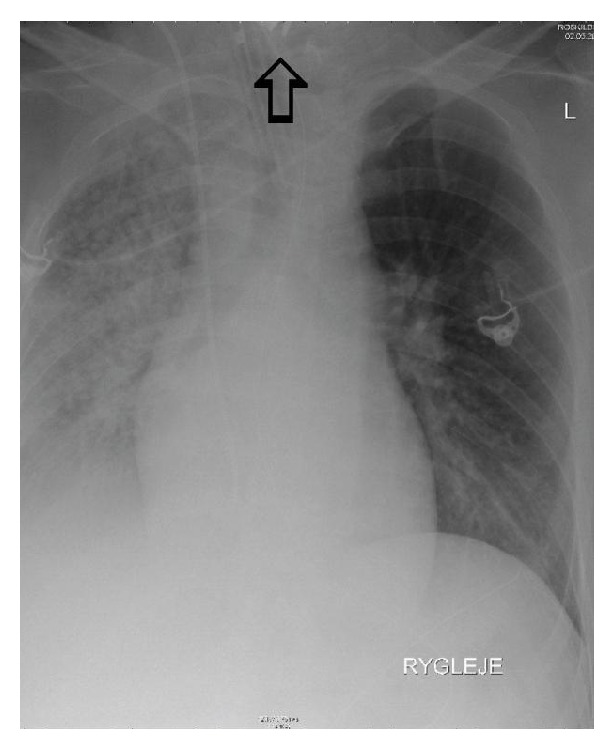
Chest X-ray 6 months prior to admittance to our department. Severe right-sided pneumonia and a suspected foreign object in the oesophagus.
